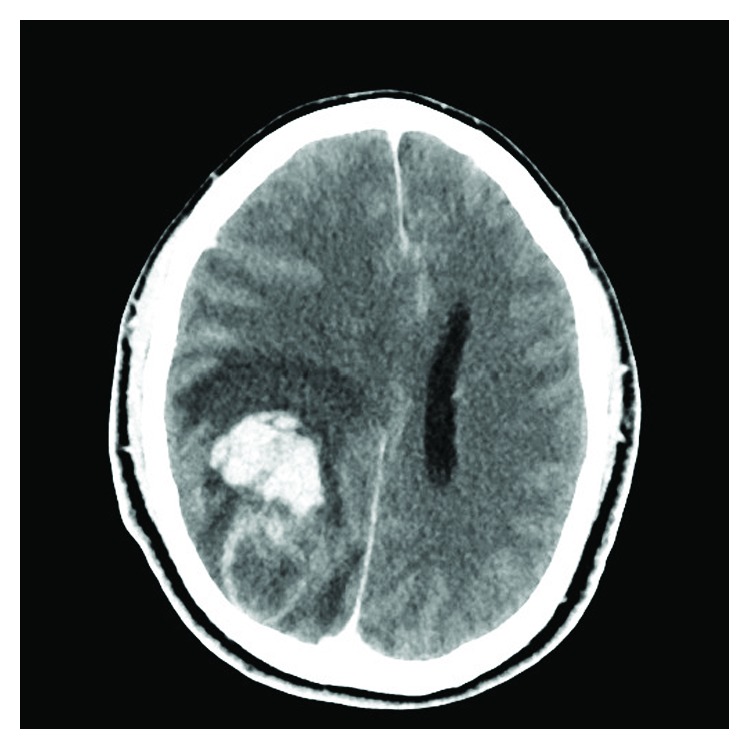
Preoperative post contrast-enhanced axial cut of the CT scan of the brain showing tumor masses located parieto-occipitally in the right hemisphere surrounded by perifocal edema and a 54 × 31 mm intraparenchymatous bleeding with affection of midline structures.
